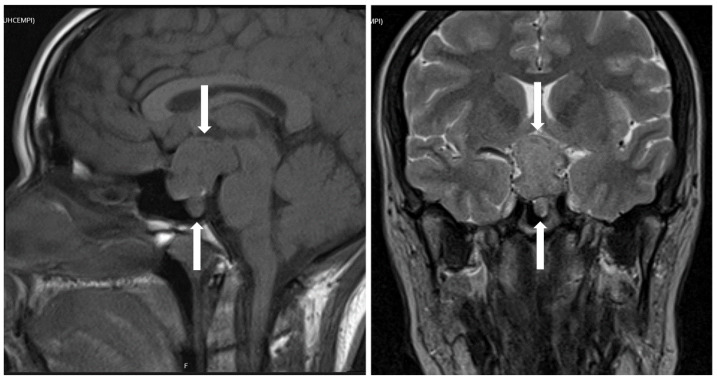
Magnetic resonance imaging of sparsely granulated somatotroph tumor. The pituitary tumor has expanded upwards to compress the optic chiasm and infiltrated downwards through the floor of the sella turcica into the sphenoid sinus (straight arrows, right and left). On T2 weighted imaging this lesion is hyperintense.
